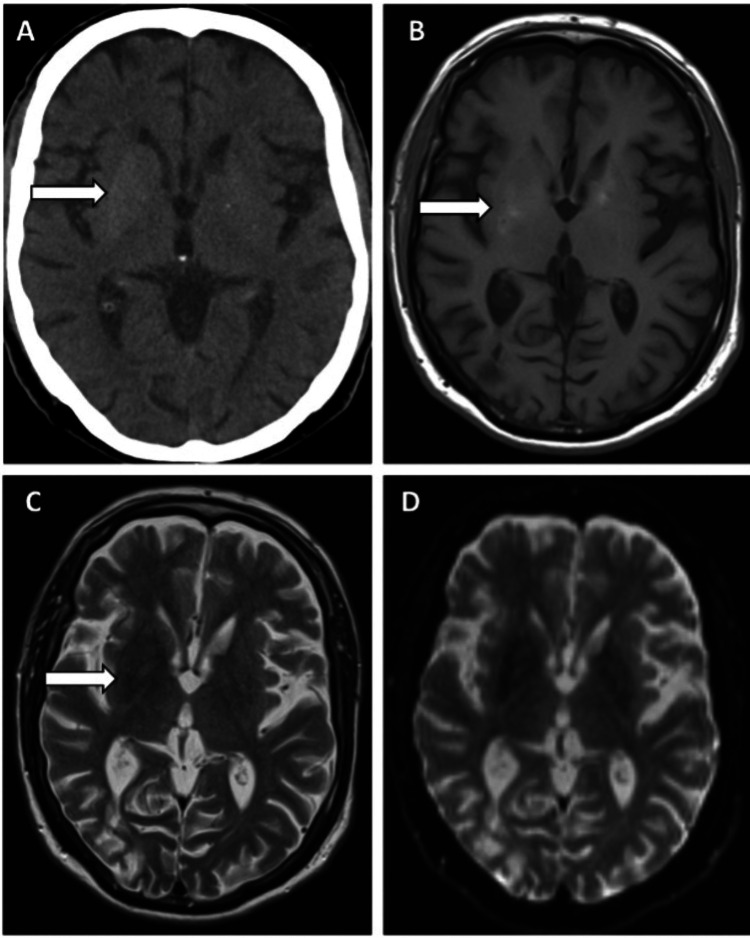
Brain images of case 1. A. Axial noncontrasted brain CT scan on admission demonstrates faint and homogeneous increased density of the right lentiform nucleus (LN; arrow). This density alteration respects the expected anatomy being confined to the LN without marked distortion. No signs of mass effect or edema. In the remaining parenchyma, there were no signs of acute intracranial lesions.
